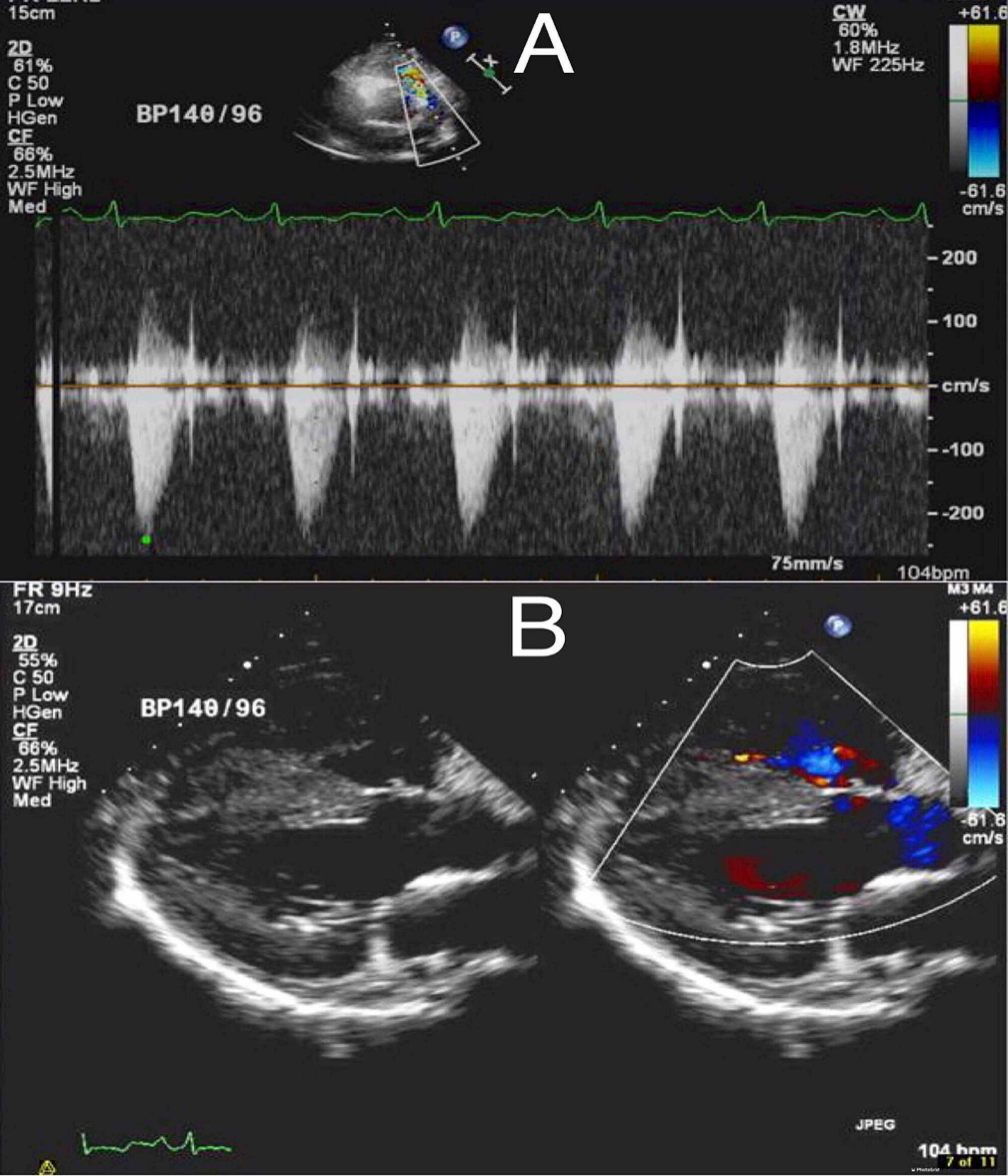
Patient’s post operational echocardiogram demonstrating (A) prosthetic pulmonary valve and (B) non-leaking ventricular septal defect closure.
